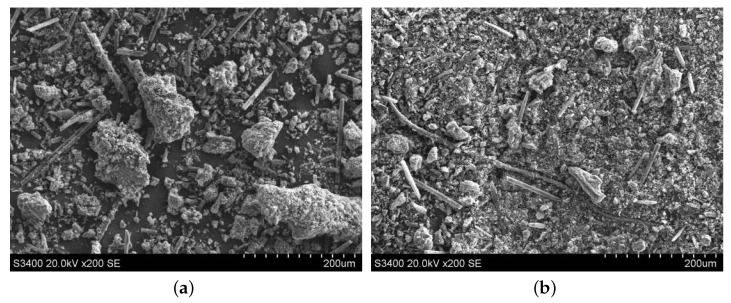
Morphologies comparison when $a_{p}$ is 0.5 mm. (a) is ordinary cutting and (b) is ultrasound- assisted cutting.
